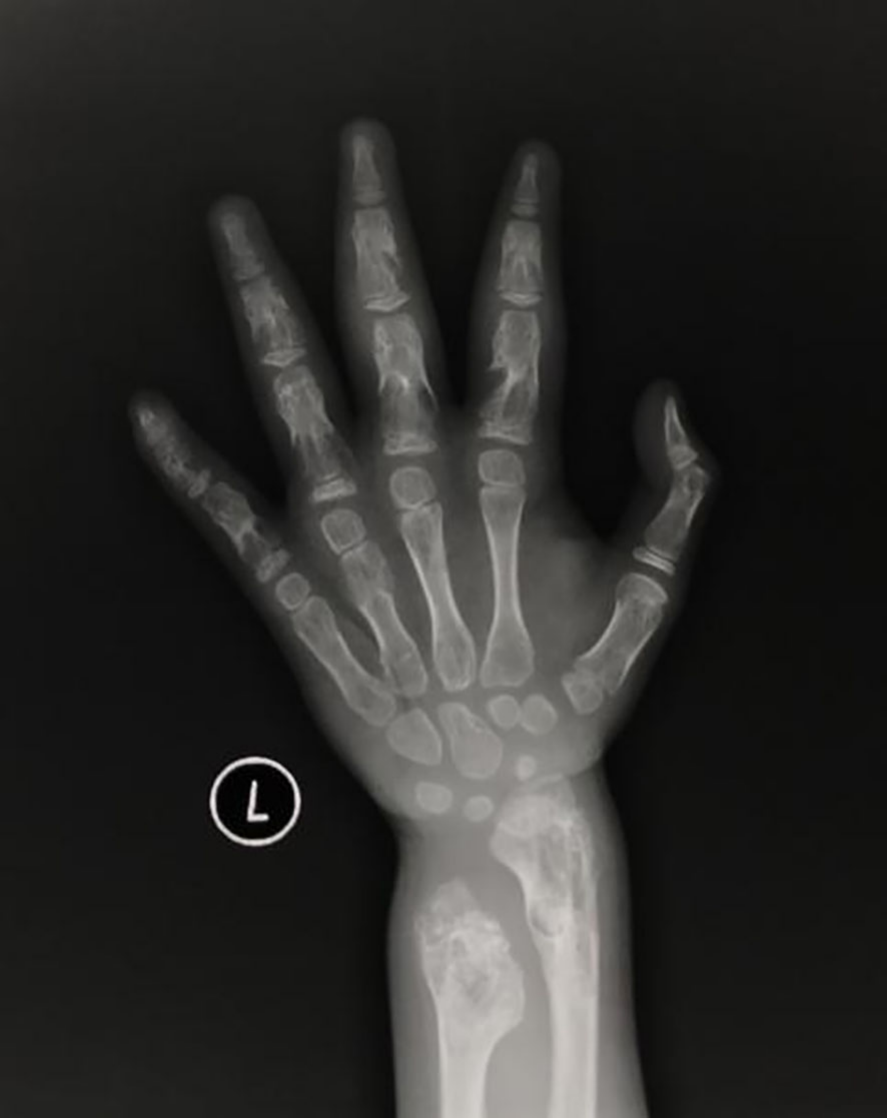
Plain bone X-ray showed multiple lytic bone lesions in left ulnar, radius and phalanges.
